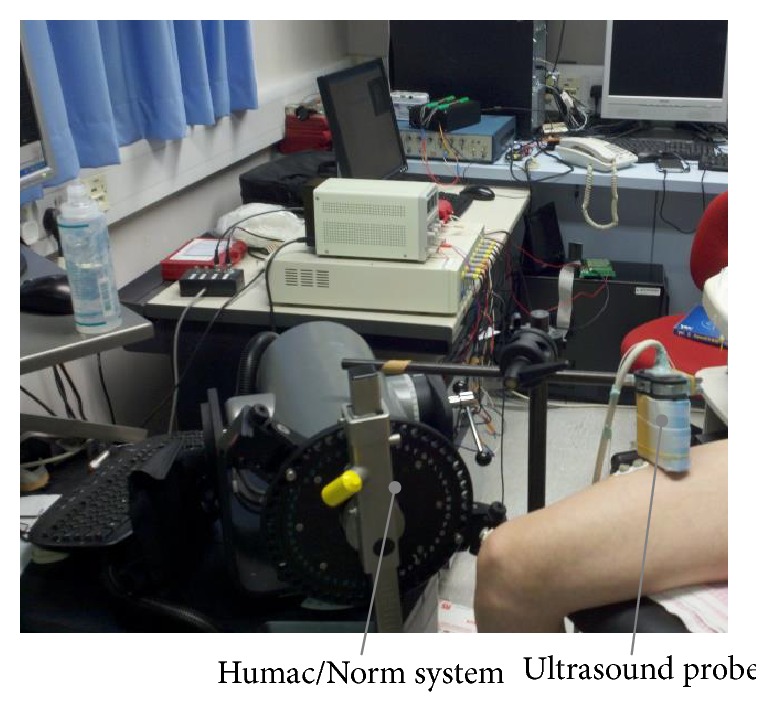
Experimental setup including the torque and ultrasound image data collection modules.
